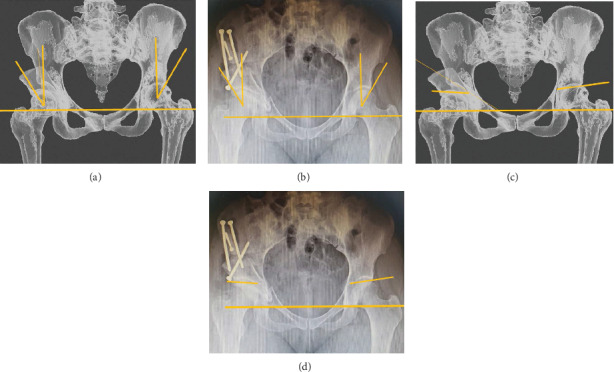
Measurement of the center edge angle (a and b) and the Tönnis angle (c and d), for both the planned (a and c) and actual radiographs (b and d). The obtained center edge angle is equal to as planned, and the Tönnis angle is 2° less than as planned.
